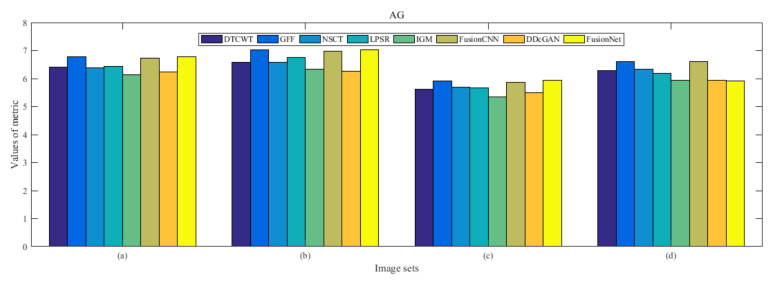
Values of average gradient (AG) in the fused images (MRI-CBF): (a) is composed of eight images in Figure 42, (b) is composed of eight images in Figure 43, (c) is composed of eight images in Figure 44, (d) is composed of eight images in Figure 45.
